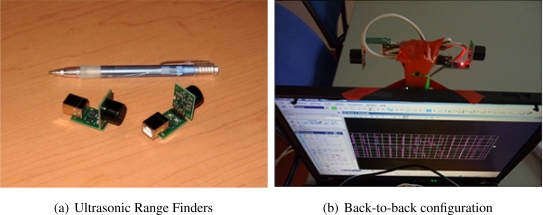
(a) The two ultrasound range sensors connected to the USB interface modules. (b) The two sensors in a back-to-back configuration installed on top of the portable computer carried by the user.
